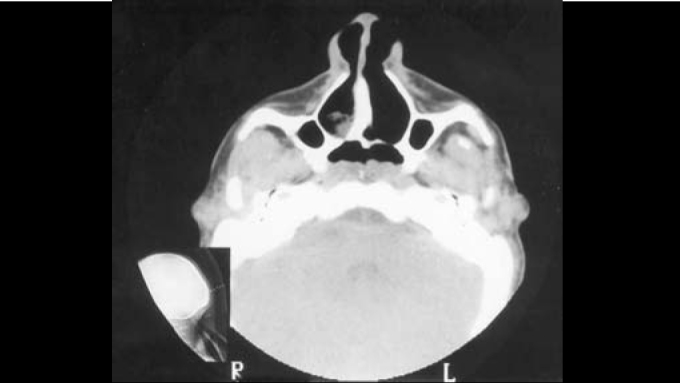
CT scan showing choanal atresia (predominance of bone tissue on the right lamina) –axial section.
